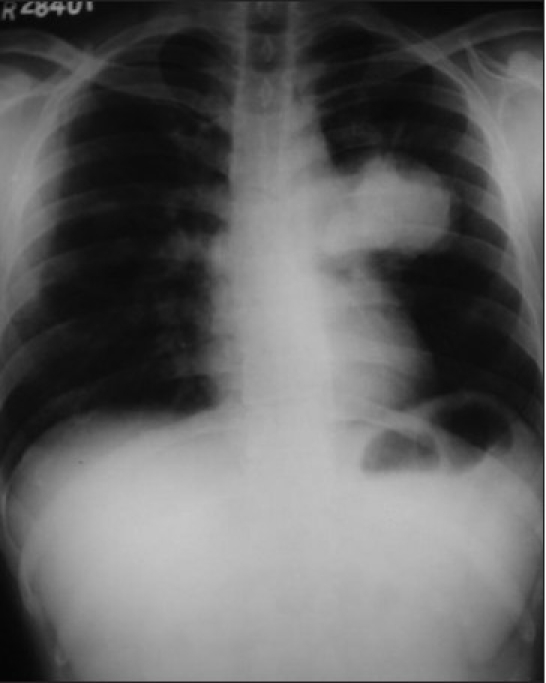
Chest radiograph showing large well defined mass near the left hilum
